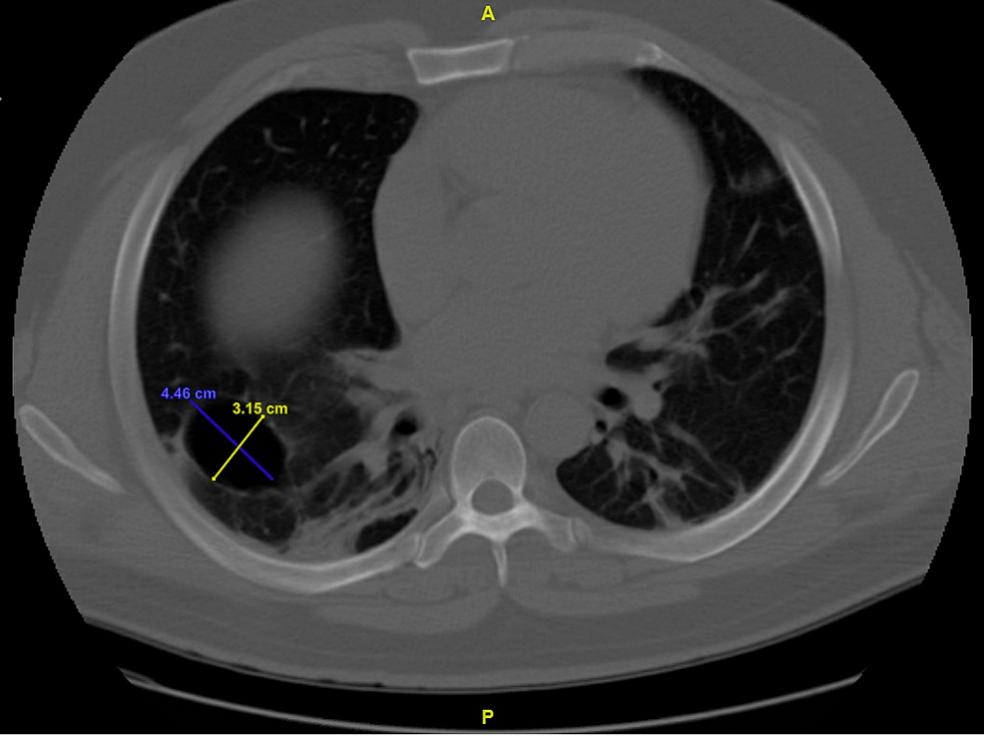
Computed tomography of the chest without contrast demonstrating improvement in overall size and wall thickness of cystic lung lesion along right major fissure at two-month follow-up, measuring 4.5 x 3.2 cm.
